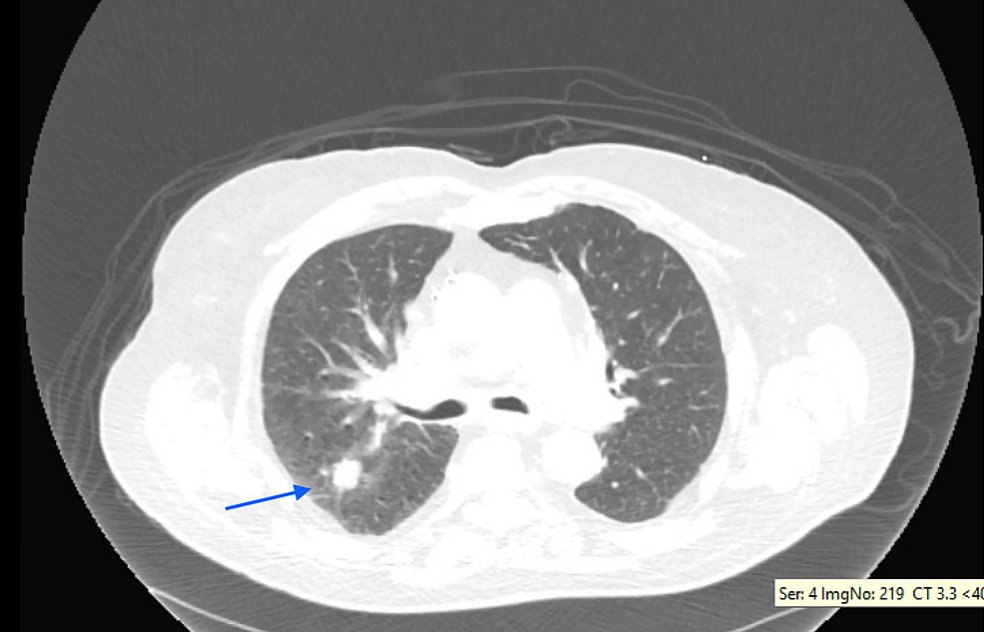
CT chest (lung window) Right upper lobe nodule (blue arrow)
